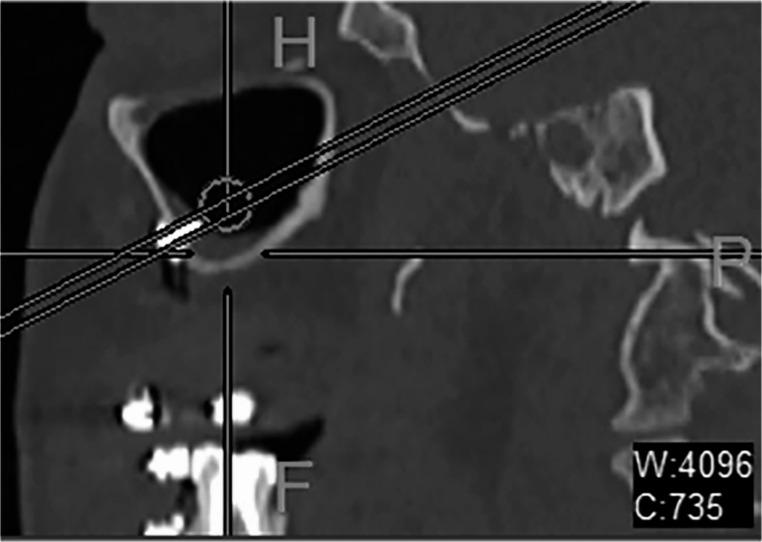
Postoperative CT scan showing measurement of screw length in the piriform aperture and zygomatic buttress
